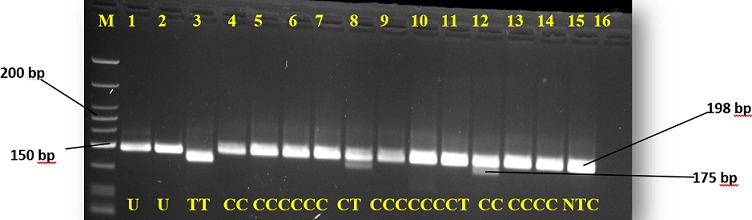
Agarose gel representation of C677T genotyping; M represents the DNA marker (50-700 bp); lanes 1 and 2 are undigested PCR products (198 bp); lane 3 shows TT genotype (175bp); lanes 4-7, 9-11, and 13-15 show the CC genotype (198 bp); lanes 8 and 12 show CT genotype (198 and 175 bp)
